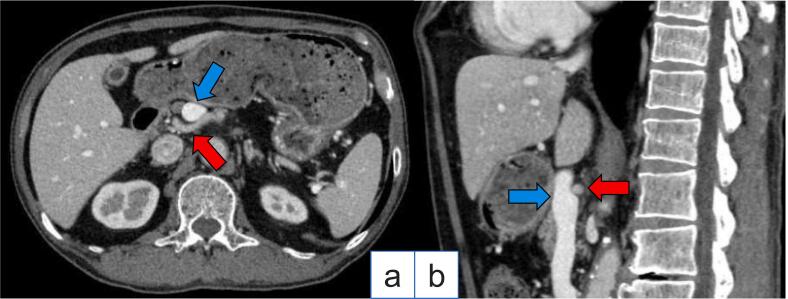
Images of preoperative abdominal computed tomography (CT). a - Axial view. Vascular anomalies with common hepatic artery (the red arrow) passing behind the portal vein (the blue arrow). b - Sagittal view. (For interpretation of the references to color in this figure legend, the reader is referred to the web version of this article.)
